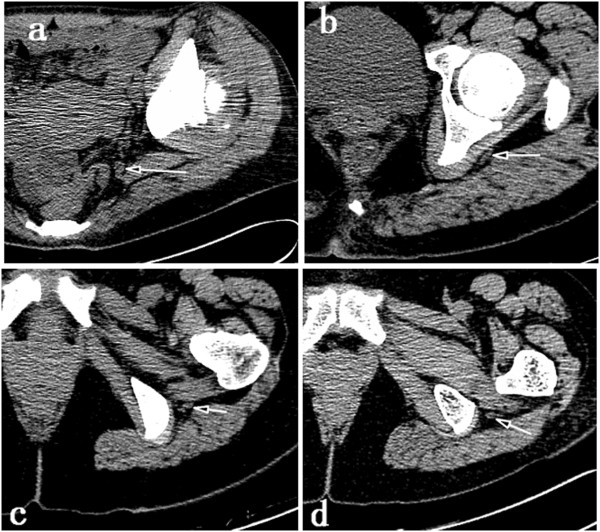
Axial CT scans demonstrating the planes used for evaluation of the sciatic nerve (indicated by arrow) in a cranio-caudal direction. The sciatic nerve was evaluated in planes perpendicular to the spine and crossing the superior border of the femoral head (a), the superior border of the greater trochanter (b), the inferior border of the greater trochanter (c), and the centre of the lesser trochanter (d).
